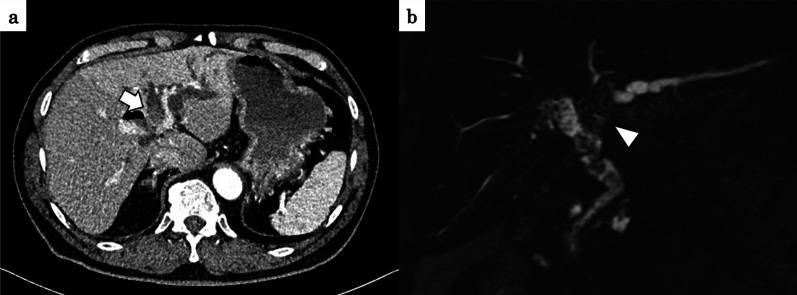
Preoperative imaging examinations of case 2. a Enhanced CT imaging showed tumor with a slight enhancement in the intrahepatic left bile ducts (white arrow). b Magnetic resonance cholangio-pancreatgraphy (MRCP) revealed a defect lesion from the common bile duct to the left bile duct (arrowhead)
